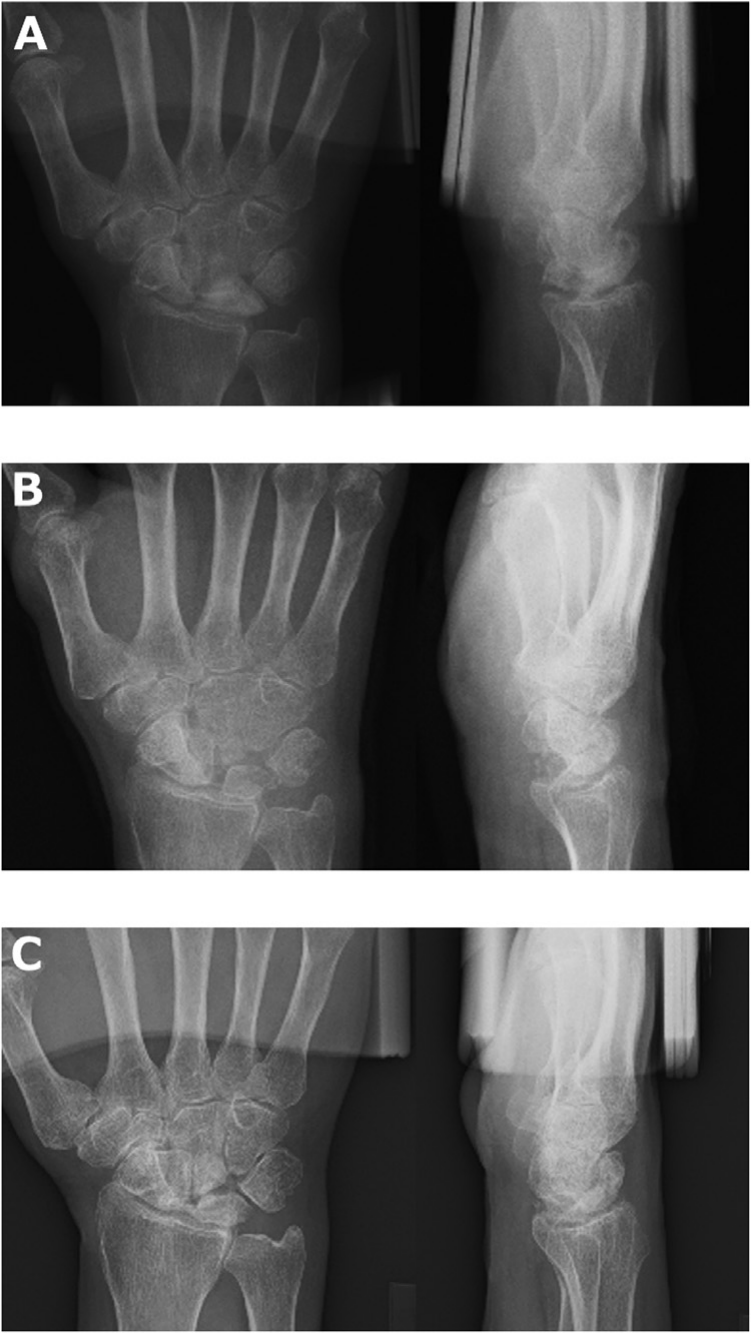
Radiograph of the right wrist of patient no. 5, a 67-year-old woman with stage IIIb Kienböck's disease. Six years and 1 month postoperatively, a radiographic change in and around the pisiform was found, especially between the capitate and the transferred pisiform. The patient experienced decreased pain, ranging from severe to mild at the last follow-up. A) Before surgery, B) immediately after surgery, C) 6 years and 1 month after surgery.
